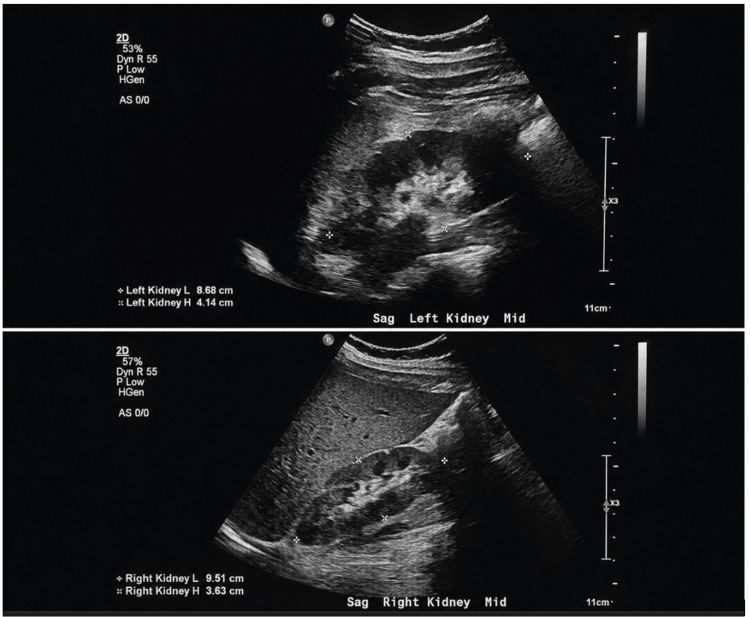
Left and right renal ultrasounds demonstrating normal bilateral kidney morphology
